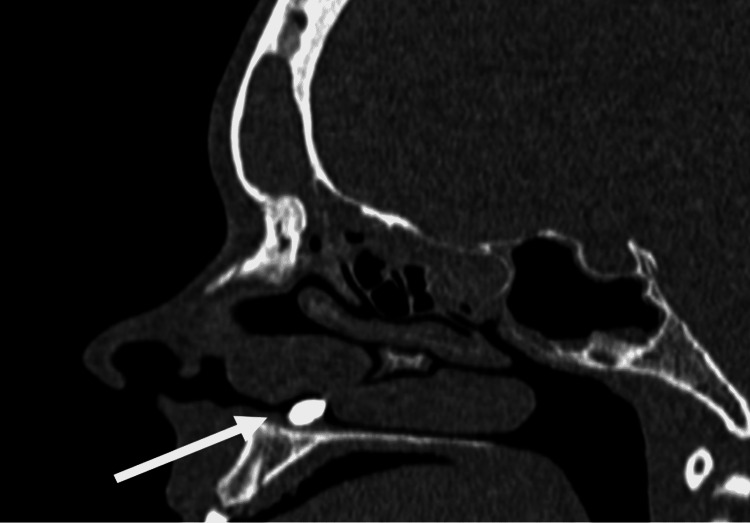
Preoperative sagittal computed tomography imaging of the paranasal sinuses showing an intranasal tooth (white arrow).
